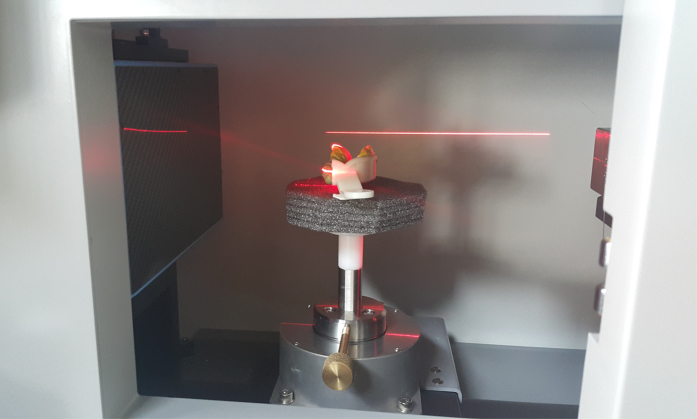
Scan method to minimize the cone-beam artifact.
